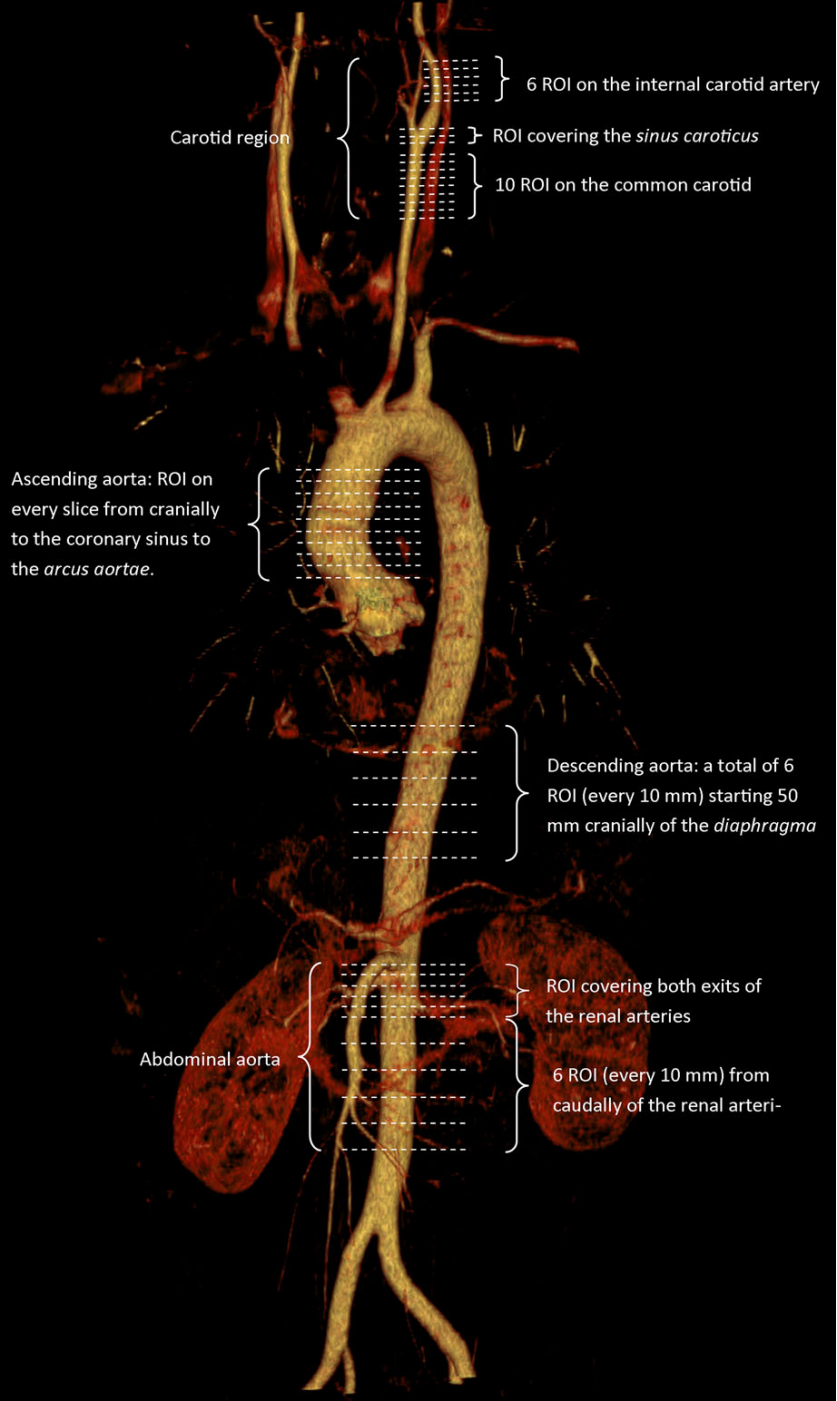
CT-angiography showing the areas of the arterial tree where regions of interest (ROI) were drawn for the analysis of FDG uptake. Slice thickness on CT was 2 mm, but distances on this image are not to actual scale
